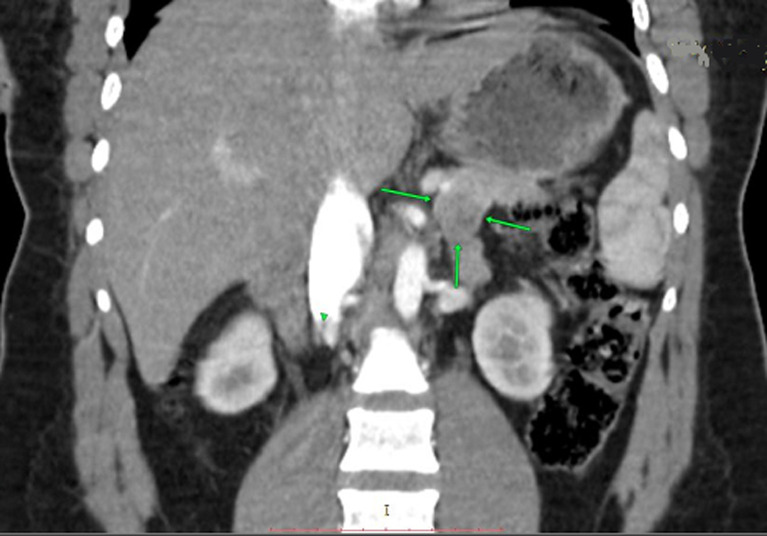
computed tomography, coronal view of the tumour
